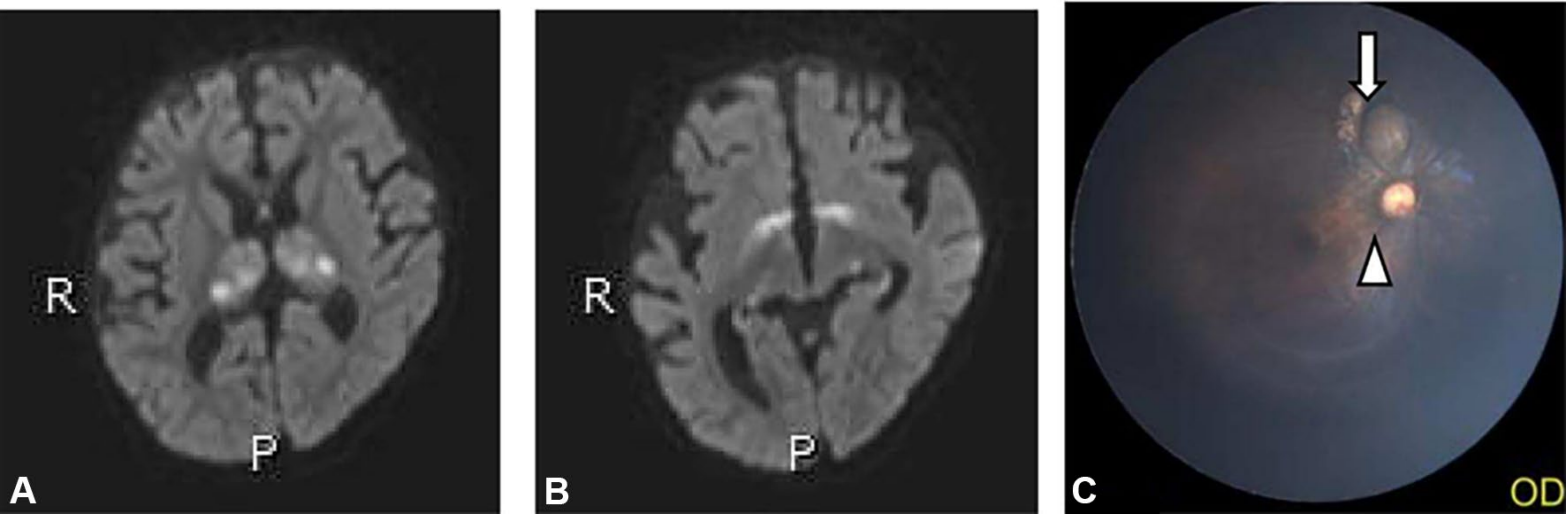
The female patient with West syndrome, was started on VGB at a dose of 75.0 mg/kg per day at the age of six months, with a cumulative VGB dose of approximately 141.5 g. A. DWI sequence showed bilateral thalamic symmetrical hyper-intensity, heterogeneous. B. DWI sequences showed abnormal hyper-intensity in the bilateral symmetric globus pallidum, medial geniculate body, and lateral geniculate body with slightly less uniform signal. C. Long arrows refer to retinopathy, and triangles refer to the optic disc.
